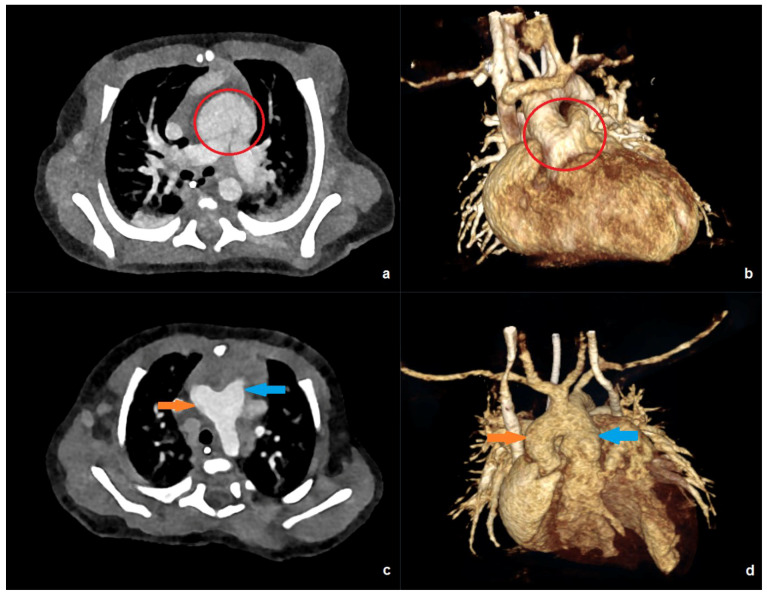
PC-CT of a 10-day-old patient with an effective dose of 0.19 mSv: (a) transverse maximum intensity projection, and (b) 3D anterior reconstruction demonstrating a common arterial trunk (red circle). PC-CT of a 59-day-old patient with an effective dose of 0.41 mSv: (c) transverse image, and (d) 3D anterior reconstruction showing a large aorto-pulmonary window with a connection between the pulmonary trunk (blue arrow) and the ascending aorta/aortic arch (orange arrow).
